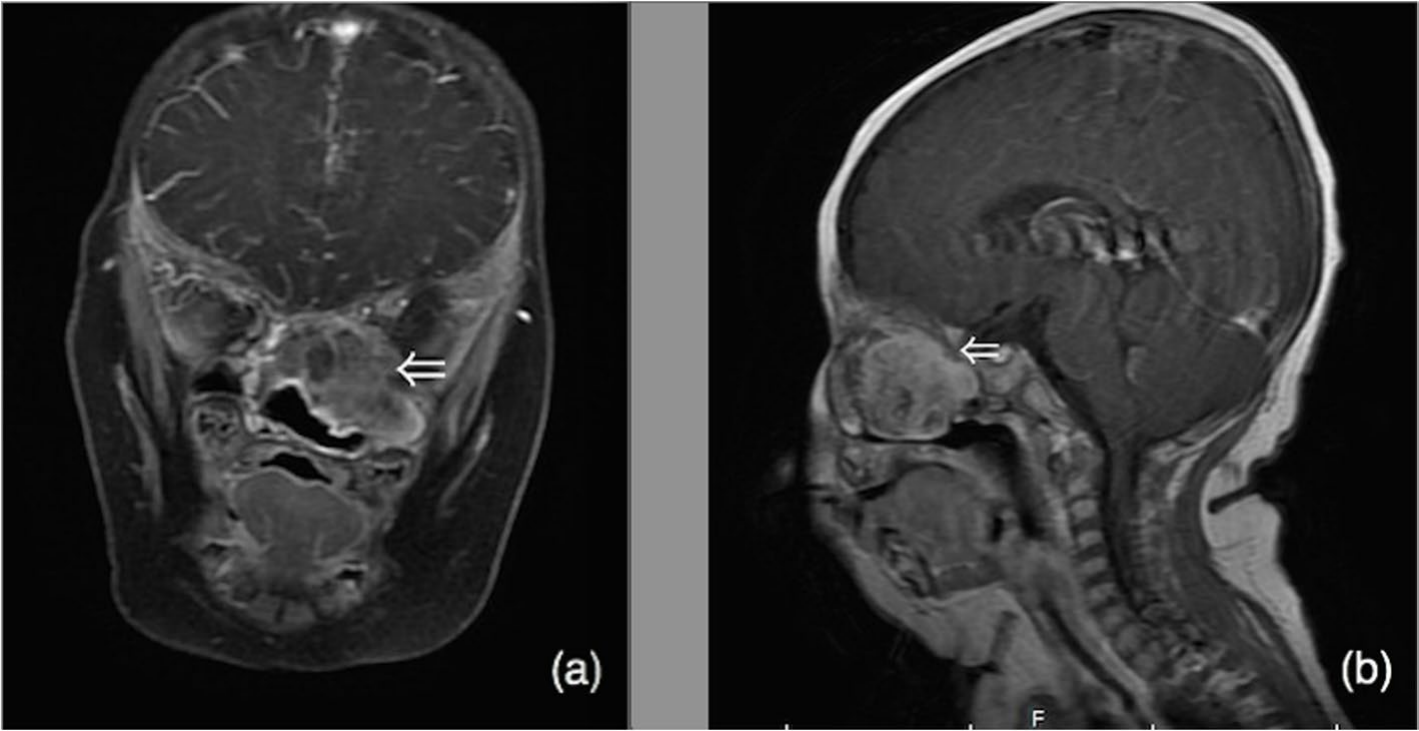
MRI image (a) Coronal post contrast T1-weighted image demonstrates left nasal mass with multiple cyst-like signals and heterogeneous enhancement. (b) Sagittal post contrast T1-weighted image showed a left nasal tumor with heterogeneous enhancement and a gap remained for ventilation in meatus nasi inferior
